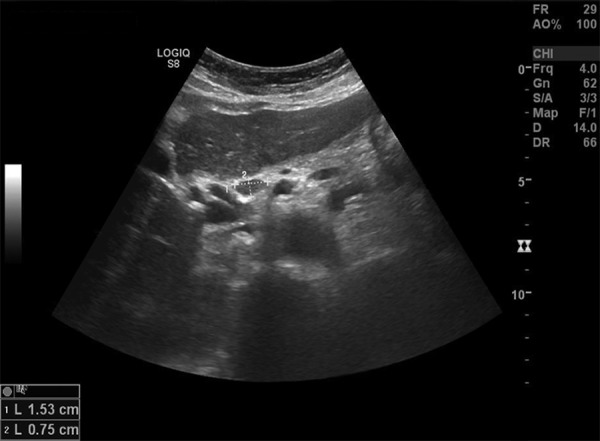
Ultrasonography of abdomen
